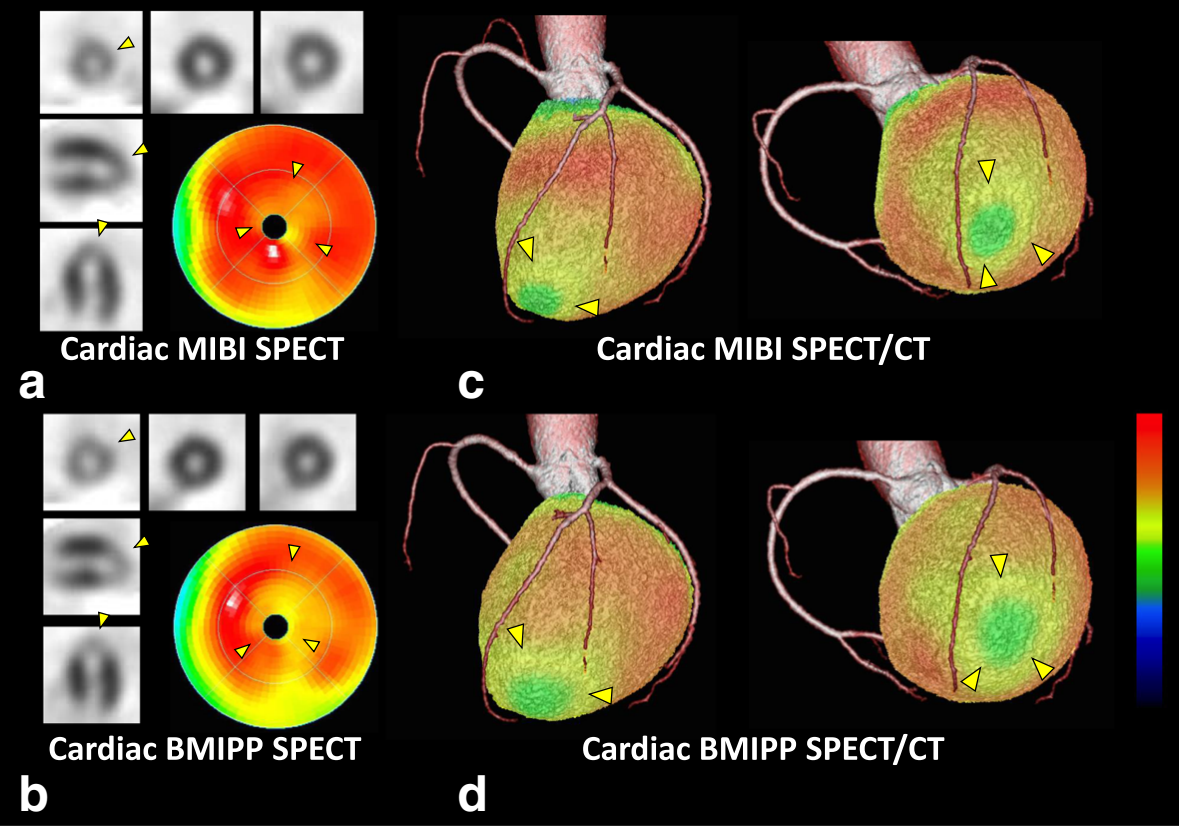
68-year-old woman with TC. Cardiac MIBI and BMIPP SPECT images show accumulation defects in the distal anterolateral to apical wall (a, b). Due to the unclear alignment of accumulation defects with coronary artery territories, SPECT-only imaging could not differentiate between TC and ACS. SPECT/CT fused images revealed that the accumulation defect extended through distal LAD and first diagonal branch territories, confirming TC (c, d)
